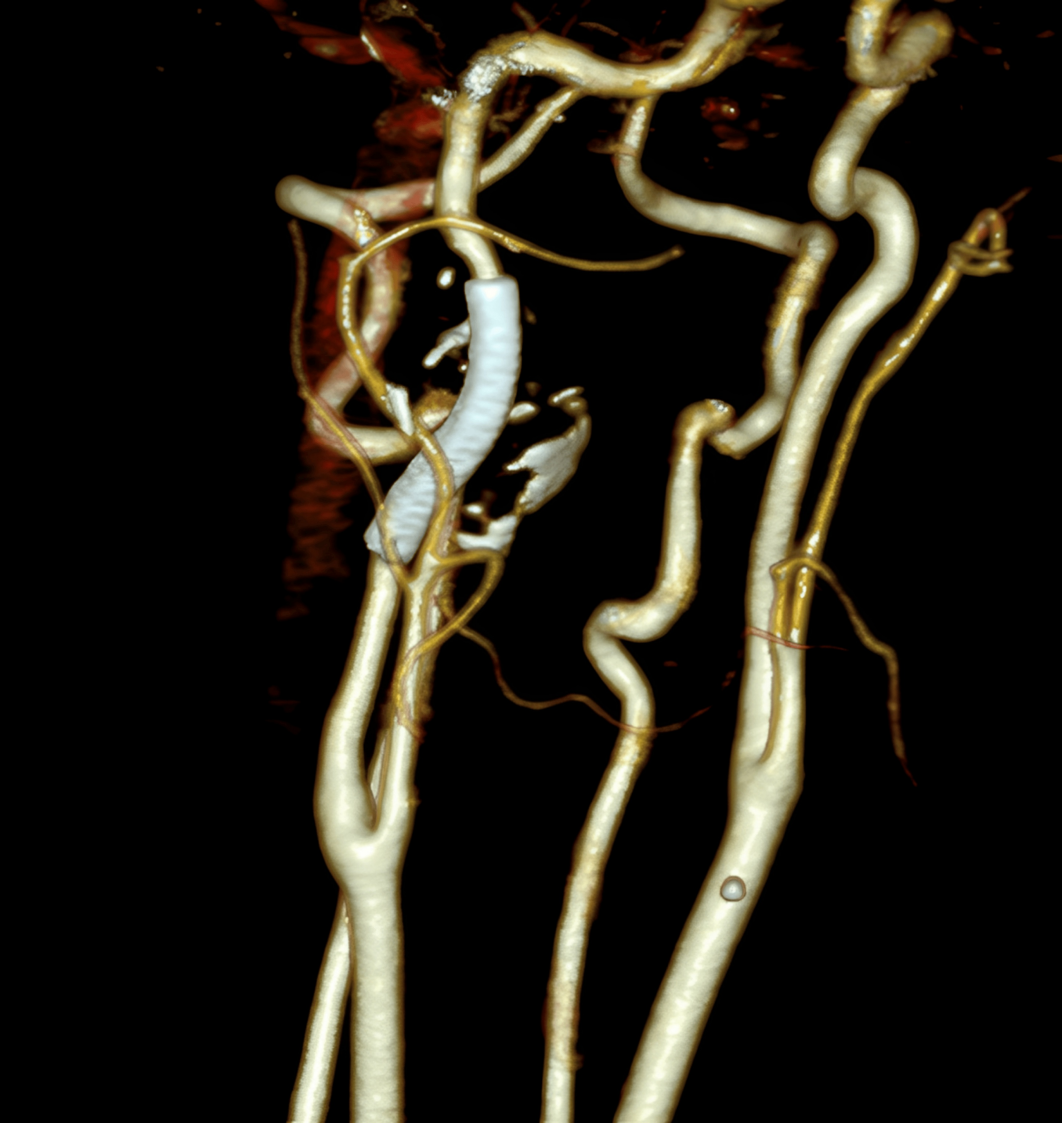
One-year CT angiography reconstruction demonstrating stent-graft patency, complete exclusion of the pseudoaneurysm, and normal distal internal carotid artery diameter
